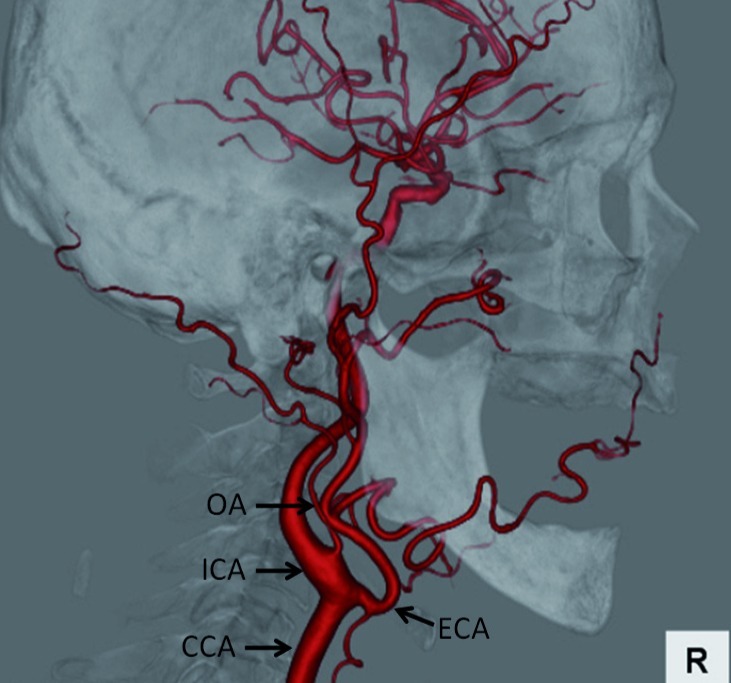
Lateral view of 3D-CTA image of the right carotid artery Abbreviations: ; CCA, Common carotid artery; ECA, External carotid artery; ICA, Internal carotid artery; OA, Occipital artery
